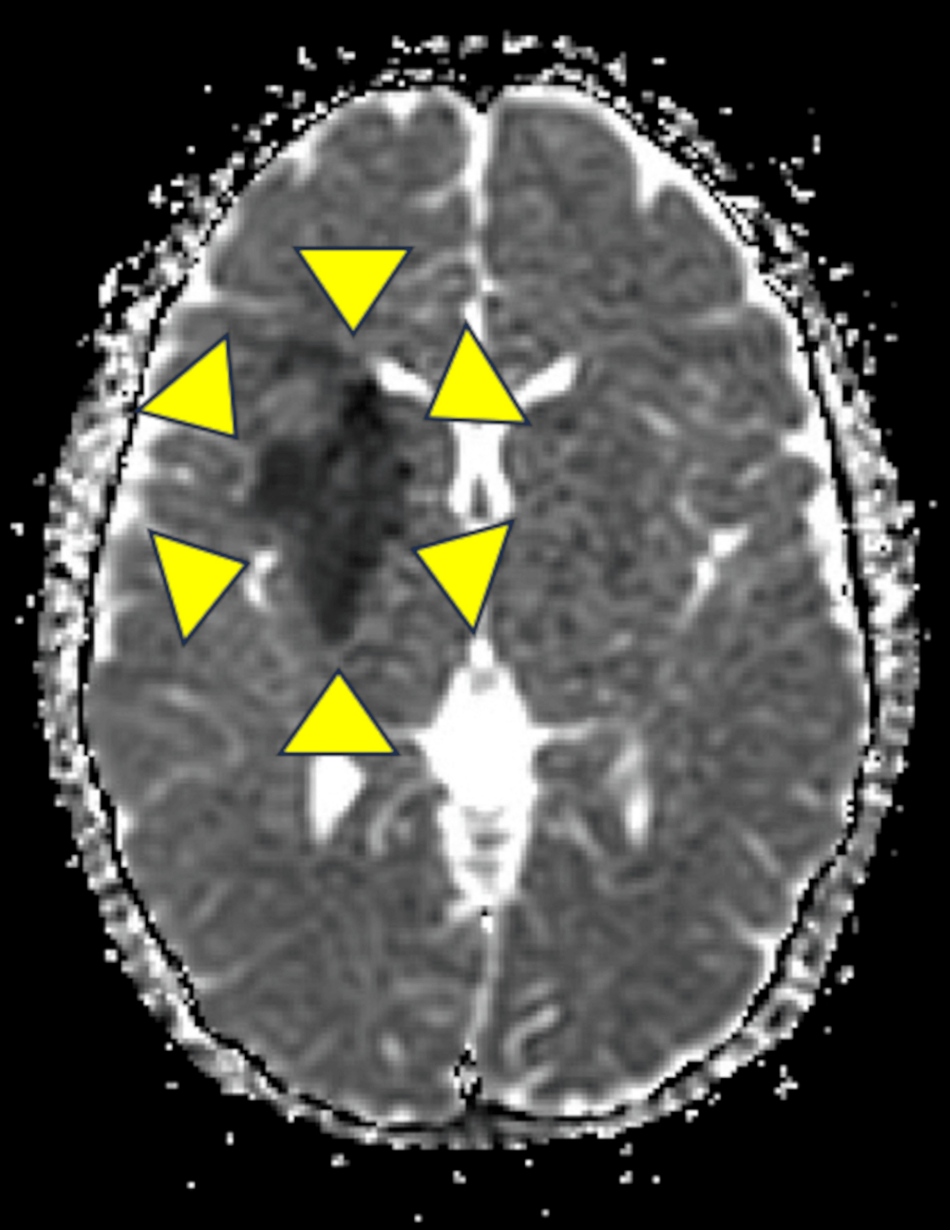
Apparent diffusion coefficient (ADC) MRI image of the head on day 2 of admission. There is an area of low signal, indicative of cerebral infarction in the right middle cerebral artery (area surrounded by yellow arrowheads).
